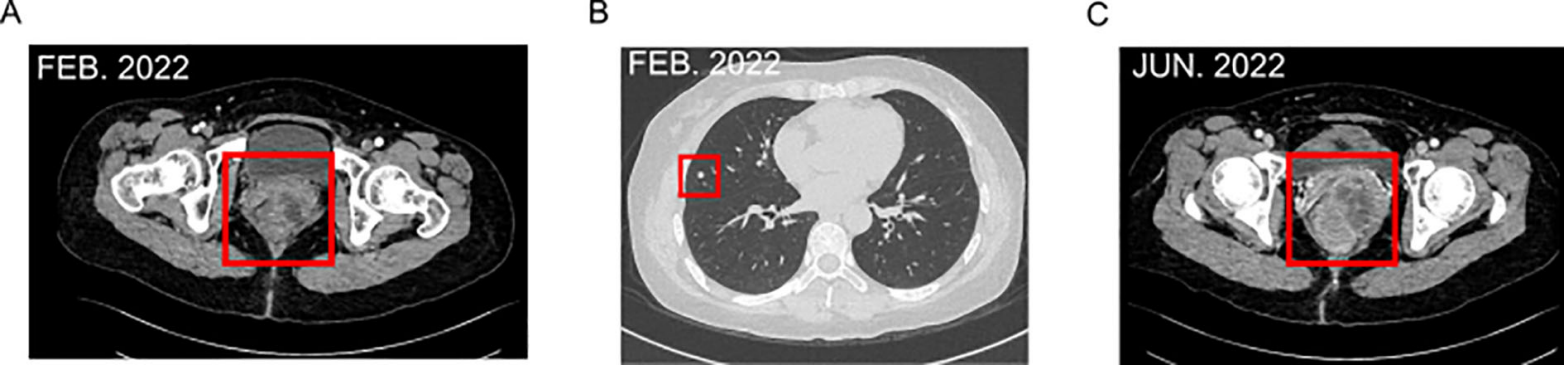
(A) Enhanced CT scan on February 17, 2022, the lower rectal lesion and pelvic lymph nodes had significantly enlarged, with invasion into the vagina. (B) Solid nodules in the right upper and middle lobes of the lung. (C) June 2022, Enhanced CT showed a reduction in rectal lesions and pelvic lymph nodes.
